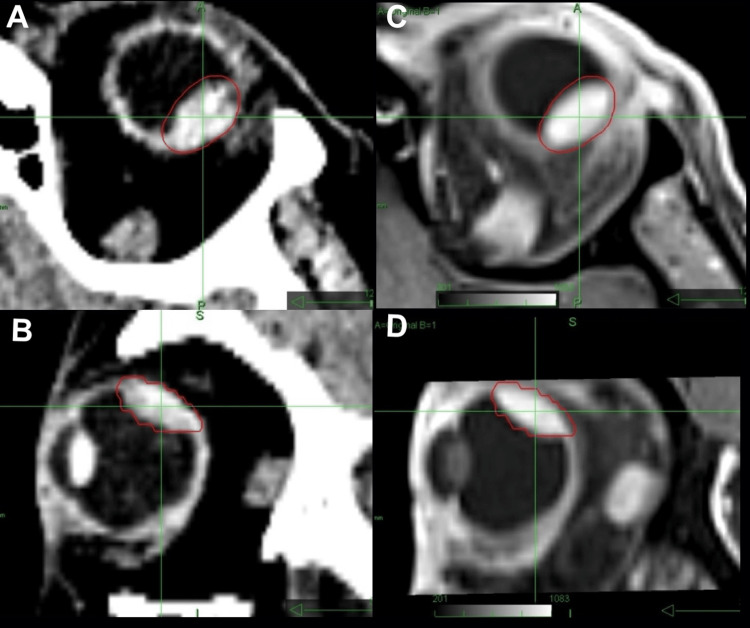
CTV: Tumor volume (red) on CT axial (A) and sagittal (B) and contrast-enhanced T1 MRI in axial view (C) and sagittal view (D). CTV: clinical target volume, MRI: magnetic resonance image.
